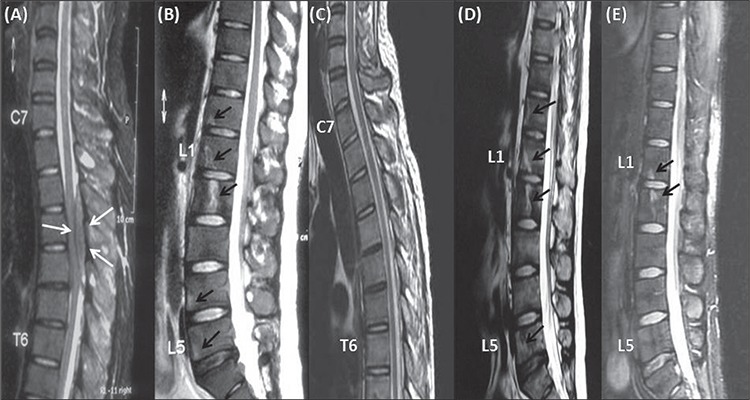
T2 weighted MRI of the patient’s spine. (A+B): Images taken at presentation showing an intraspinal mass (white arrows)located extradurally between T2 and T4 vertebral bodies with multiple hyperintense bony lesions (black arrows) on T12, L1, L2, L4and L5 vertebral bodies. (C+D): Images taken 1 month after treatment showing no evidence of intraspinal mass but the bony lesionson thoracic and lumbar vertebral bodies were persistent (black arrows). (E): Image taken after the third consolidation therapy showinga few residual bony lesions on L1 and L2 vertebral bodies (black arrows).
